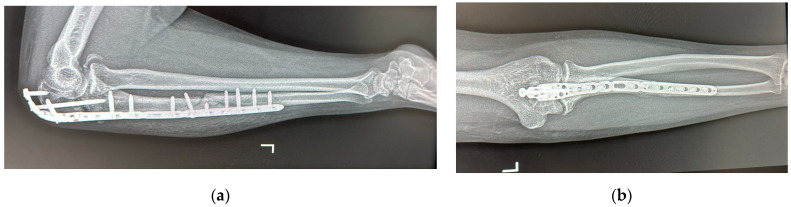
Follow-up radiograph at six weeks: (a) lateral view with elbow flexed at 90°; (b) anterio-posterior view.
